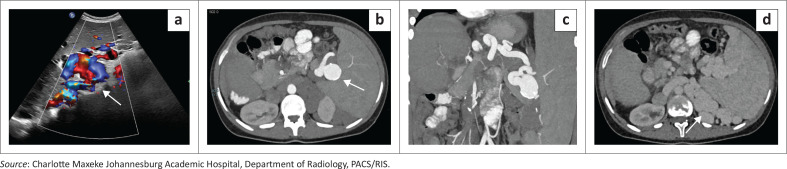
Splenic artery aneurysm in a 19-year-old patient with a complex medical history including a prior Kasai procedure and longstanding portal hypertension.(a) Colour Doppler ultrasound demonstrates a well-defined vascular lesion with the characteristic “yin–yang” bidirectional flow pattern consistent with an aneurysmal sac (arrows). (b) Axial arterial-phase contrast-enhanced CT shows a rounded saccular dilatation of the splenic artery (arrow). (c) Coronal reformatted CT confirms the aneurysm along the course of the splenic artery. (d) Axial contrast-enhanced CT demonstrates multiple serpiginous enhancing perisplenic varices (arrow) consistent with portal hypertension related collateralisation.
